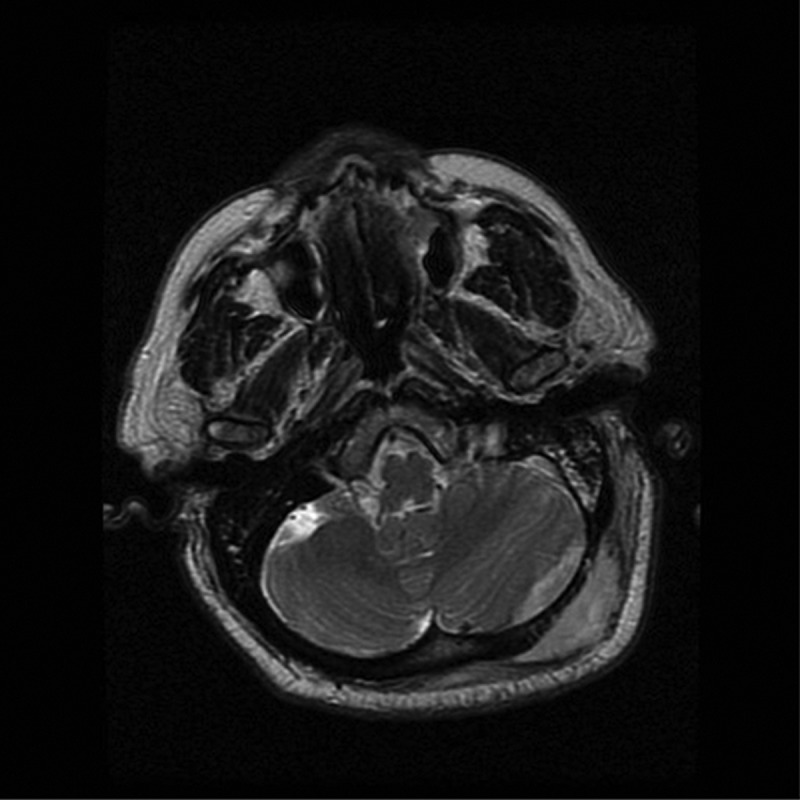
MRI axial T2 image revealing high signal intensity within left suboccipital soft tissue and epidural space.
